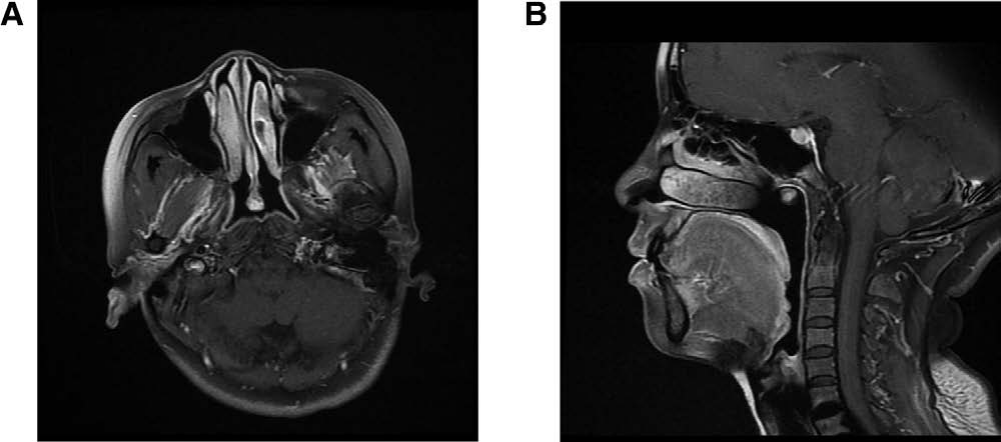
(A) MRI showed a 0.7 × 0.9 × 0.9 cm sized, heterogeneously contrasted mass arising from the posterior edge of the nasal septum. (B) MRI showed a 0.7 × 0.9 × 0.9 cm sized, heterogeneously contrasted mass located in the posterior wall of the parietal nasopharynx.
